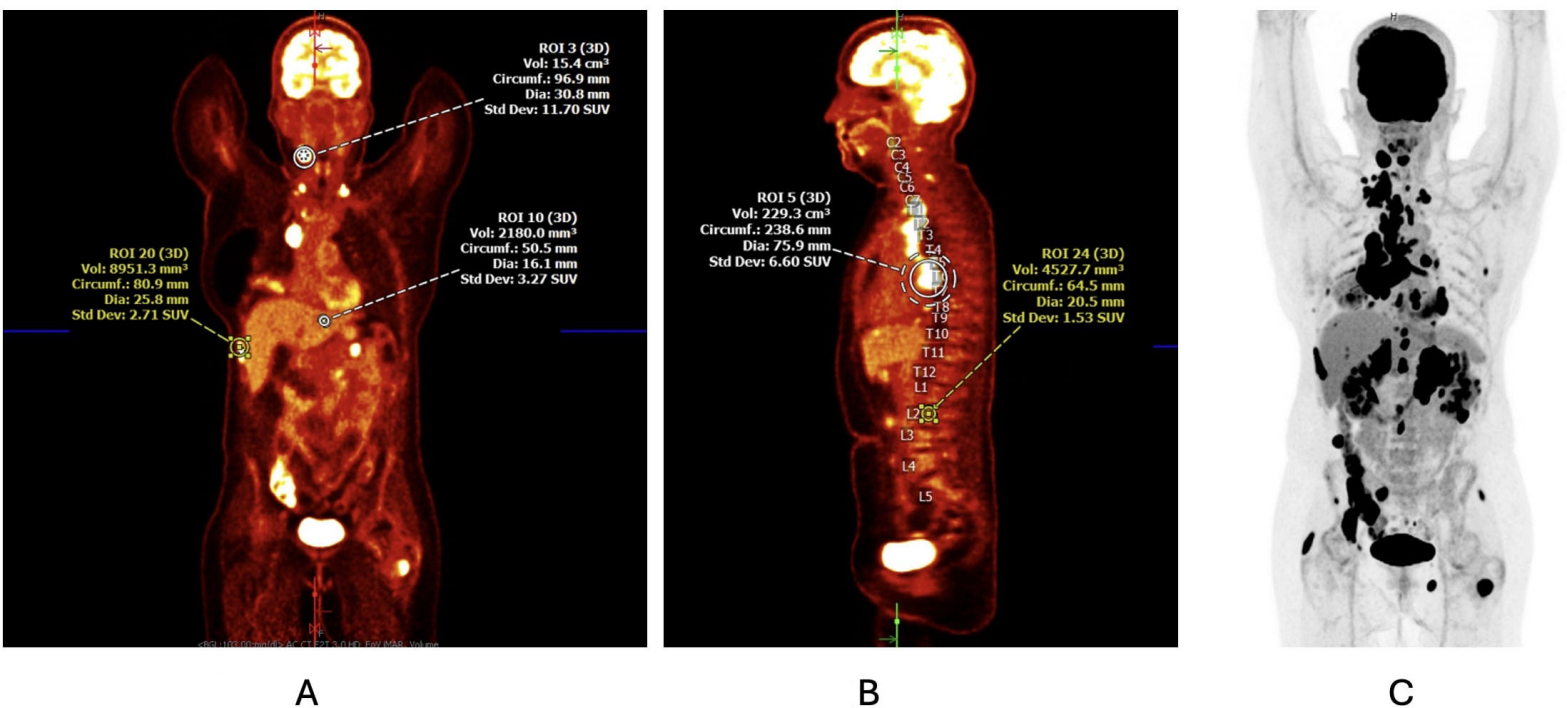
(A, B) Initial PET/CT scan at the time of diagnosis demonstrating a 6.4-cm × 4.8-cm × 7.0-cm soft tissue mass in the right perihilar region with resultant complete occlusion of the right middle lobe bronchus and right lower lobe ground-glass opacity and atelectasis. Mediastinal, paratracheal, paraesophageal, subcarinal, perihilar, and supraclavicular lymphadenopathy along with known osseous metastasis is also noted. (C) Corresponding PET scan image detailing the areas of cellular activity.
